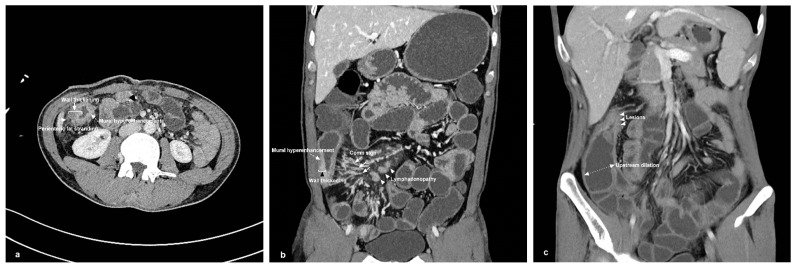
CT enterography findings in Crohn’s disease. (a) A male, 29 years old. Axial CT enterography in a patient with Crohn’s disease shows bowel wall thickening (10.9 mm), mural hyperenhancement and perienteric fat stranding (arrows); (b) Coronal CT image shows the mesenteric straight small vessels are increased, presenting as comb sign. The enlarged mesenteric lymphadenopathy, bowel wall thickening, and mural hyperenhancement also can be clearly seen (arrows); (c) A male, 41 years old. Coronal CT image shows the lesion and upstream dilation (39.7 mm) (arrows).
